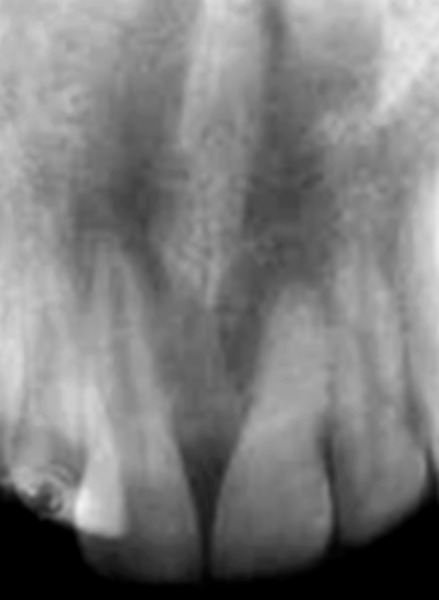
Post-treatment periapical radiograph
